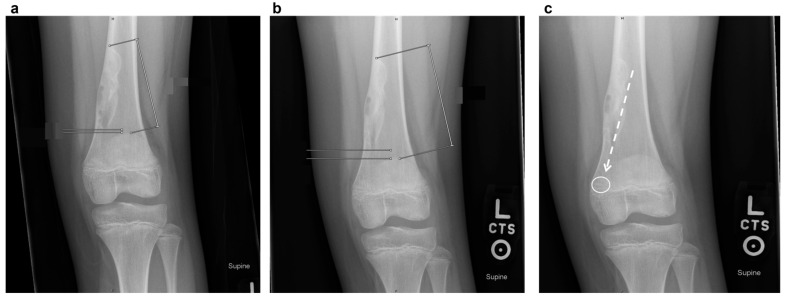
Serial radiographs demonstrating the characteristic migration pattern of NOF away from the growth plate in a 9-year-old girl over 6 months (a,b). Note the eccentric location, sclerotic rim, and “bubbly” appearance that distinguish it from other lytic lesions. Image (c) demonstrates the major axis of the non-ossifying fibroma with an imaginary line (dashed arrow) pointing toward the gastrocnemius insertion (white circle), which is a typical migration pattern for NOFs.
